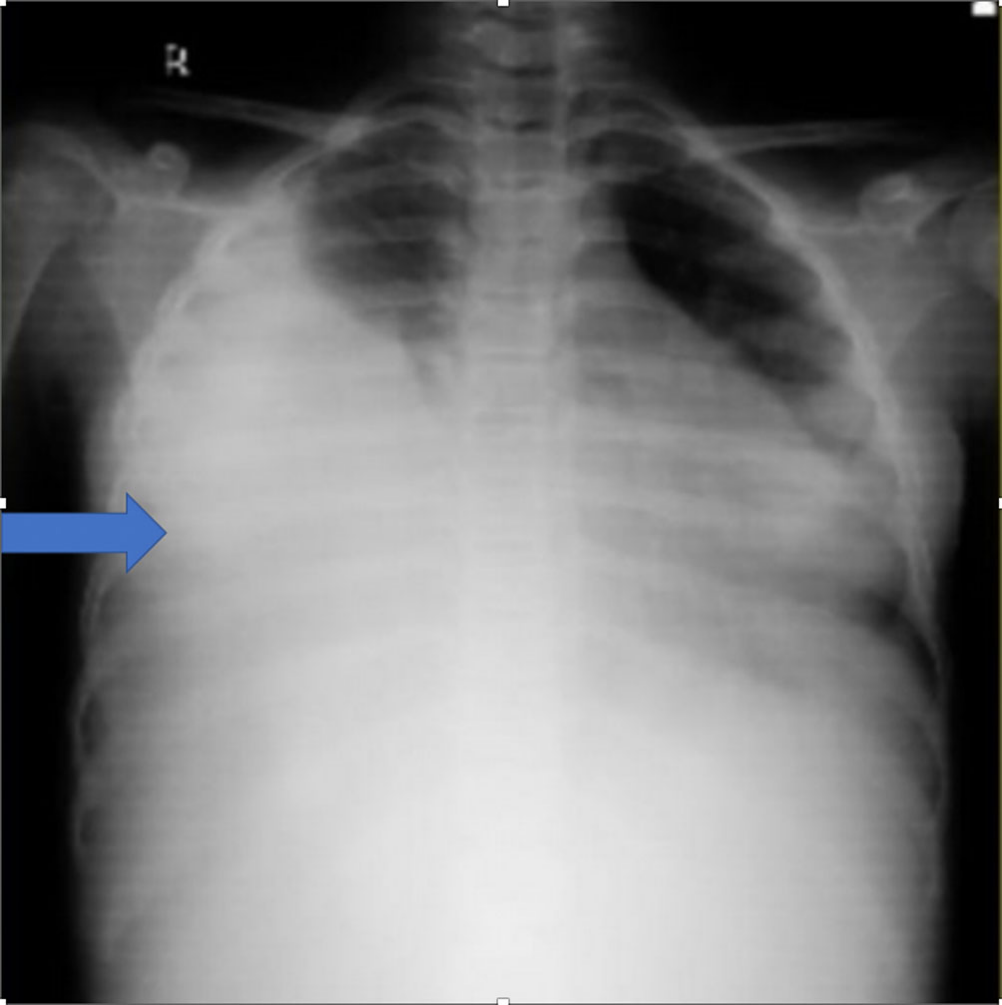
Chest X-ray showing right sided massive pleural effusion with mediastinal shift towards left.
